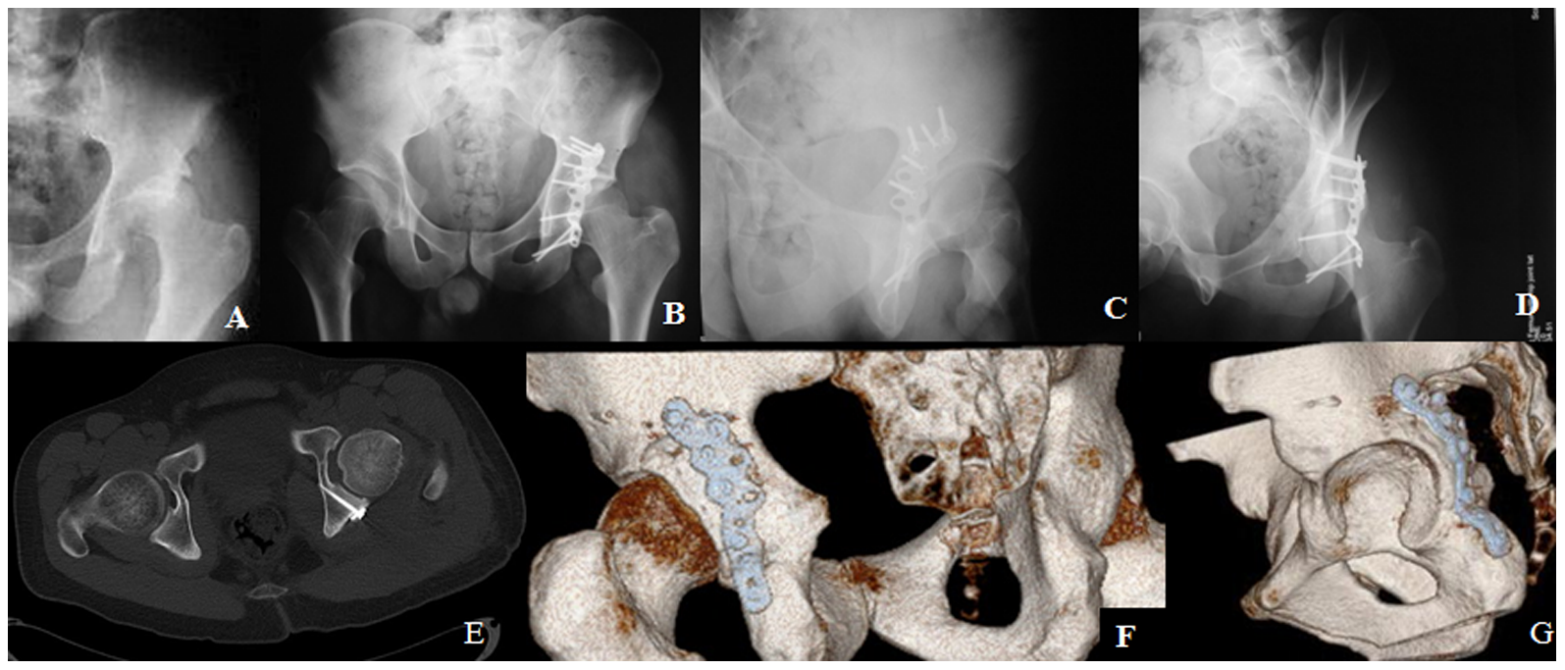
Emergency room radiograph of a 38-year-old man with a posterior wall fracture with posterior dislocation of the femoral head (A). The postoperative radiograph (B, C, D) and CT cut (E) demonstrate the extra-articular screw placement, and the VRT image shows a safe screw insertion into the posterior wall without violation into the joint (F,G).
